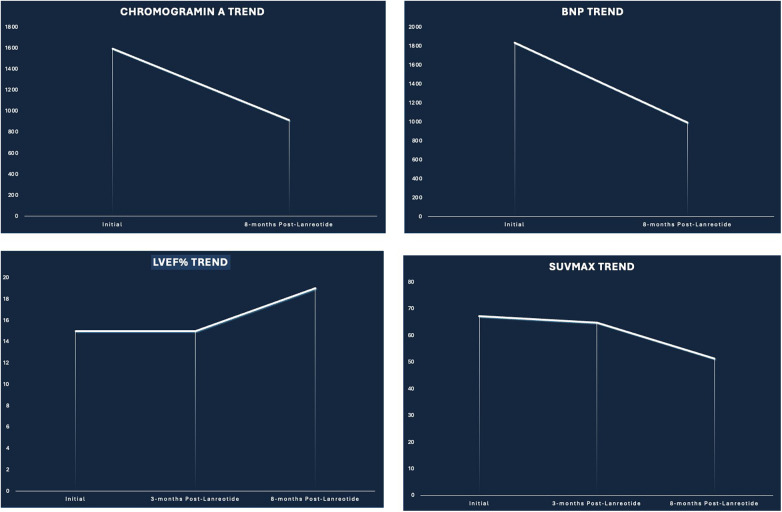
Biochemical, cardiac, and imaging response to lanreotide therapy. Legend: Trends in tumor markers, cardiac biomarkers, cardiac function, and metabolic imaging activity following initiation of lanreotide therapy. Serum chromogranin A declined from initial presentation to 8 months post-lanreotide. B-type natriuretic peptide (BNP) declined over the same interval. Left ventricular ejection fraction (LVEF%) improved initial presentation through 3 and 8 months post-lanreotide. Maximum standardized uptake value (SUVmax) on ⁶⁸Ga-DOTATATE PET/decreased at 3 and 8 months post-lanreotide relative to baseline.
